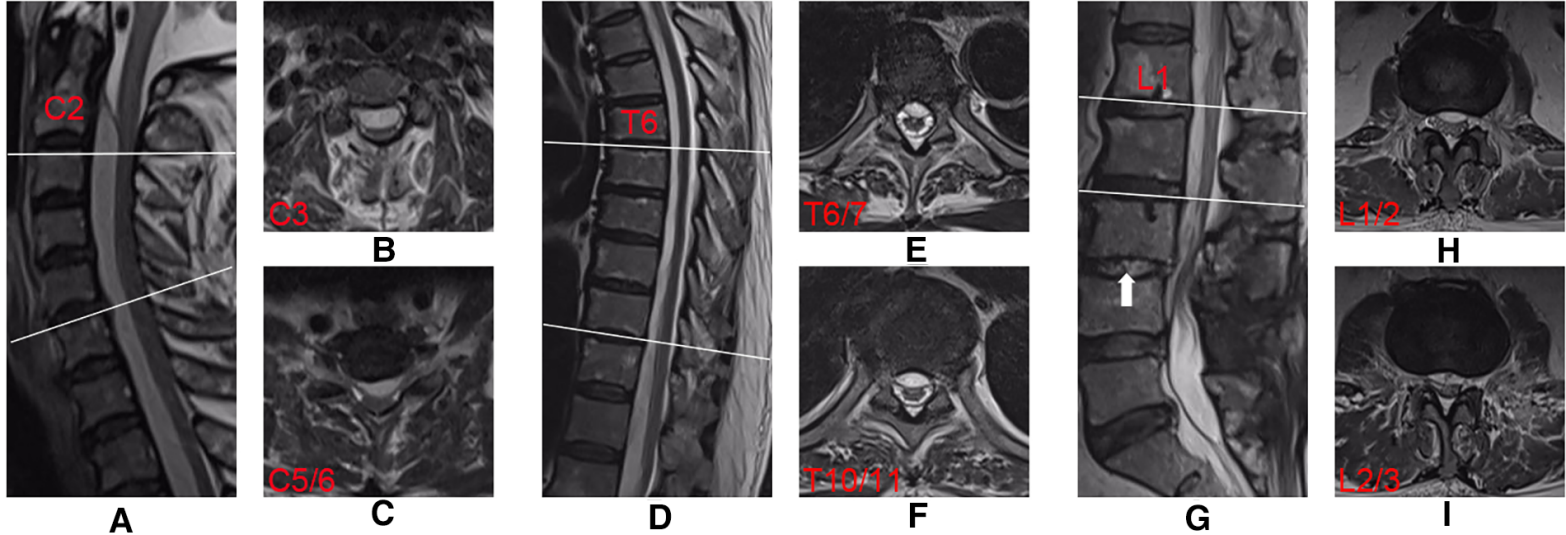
Preoperative T2-weighted MRI sequences obtained from an extensive SEA in a 70-year-old man. Midsagittal views showing the SEA (A,D,G). Axial imaging at C-3 (B), C-5/6 (C), T-6/7 (E), T-10/11 (F), L-1/2 (H) and L-2/3 (I) showing extensive ventral collection. Mixed-high signals (white arrow) on T2-weighted imaging indicate the spondylodiscitis at the L3/4 segment (G).
